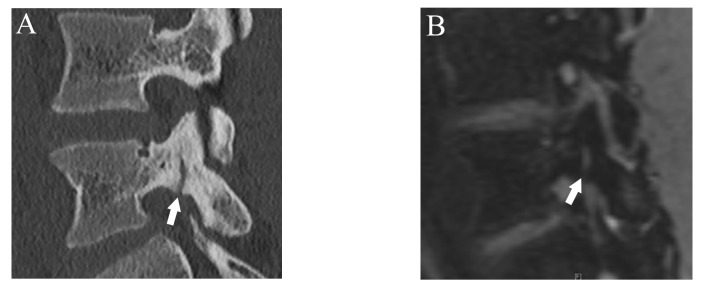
Representative images of grade 2 fracture. The left pars interarticularis of L5 from a 16-year-old male. (A) Sagittal CT image showing an incomplete but clear fracture line starting from the caudal part of the pars interarticularis (arrow). (B) Sagittal DESS image showing a linear high signal that suggests the fracture line (arrow).
